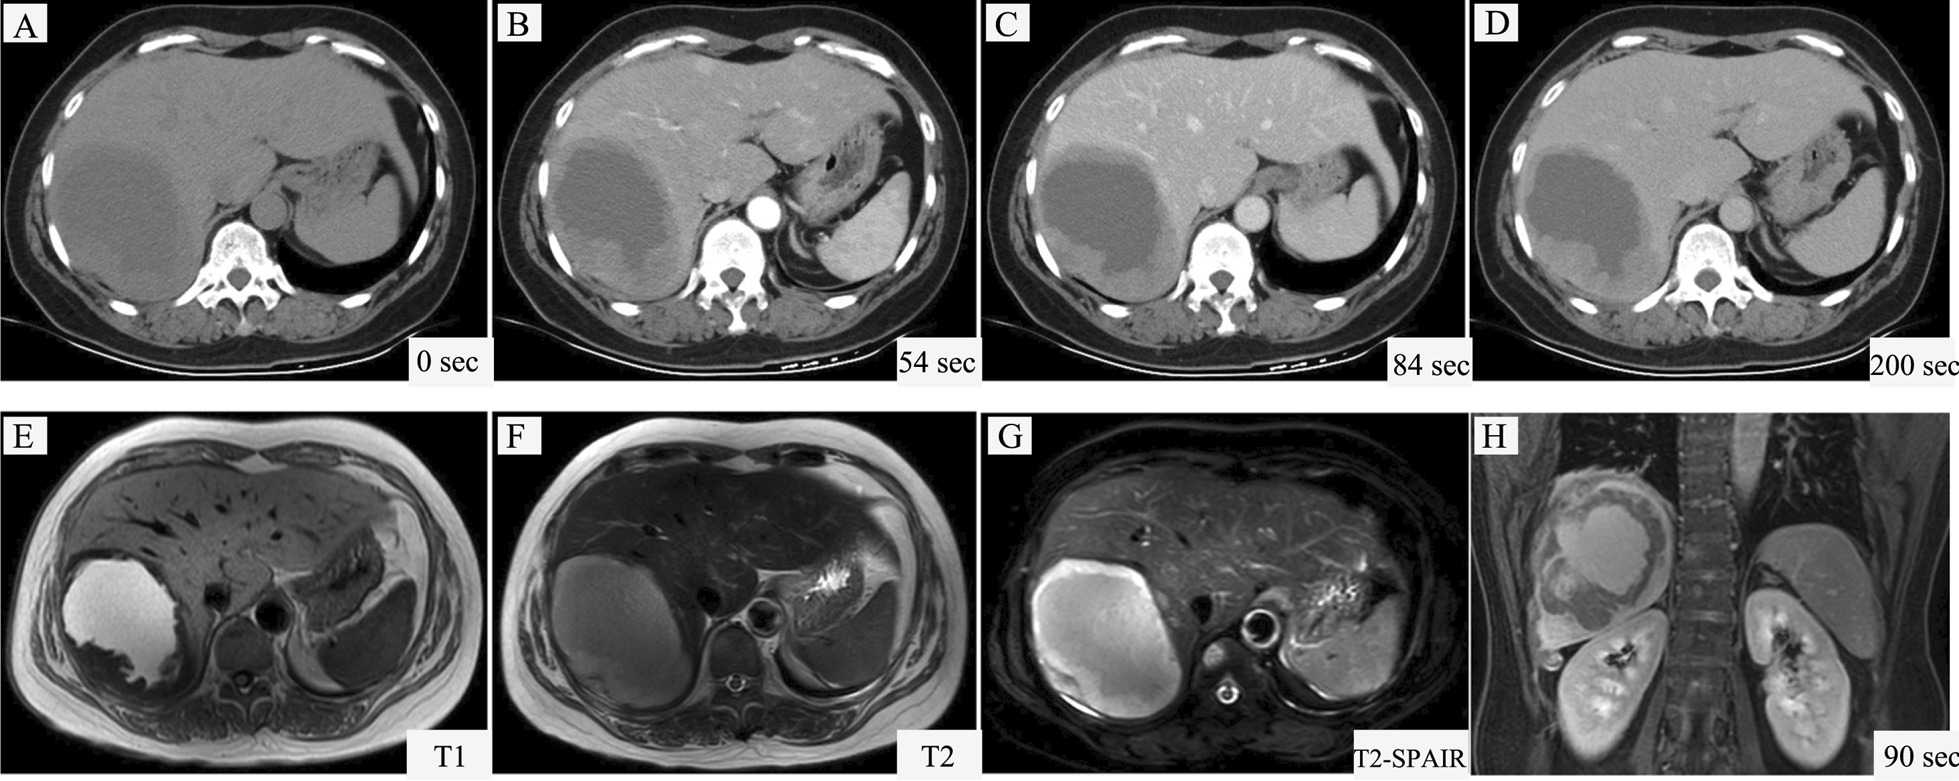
Imaging findings of the patient. Computed tomography (CT) scan showed an ill-defined, hypodense mass in the right liver lobe extending to the diaphragm (A) with moderate enhancement in the periphery of the mass at 54 s (B), 84 s (C) and 200 s (D) after intravenous injection of contrast. Magnetic resonance imaging (MRI) demonstrated an elliptic mass in the right liver lobe with an increased signal on both T1- (E) and T2- (F) images; On T2- SPAIR sequence the mass showed high signal indicating haemorrhage within the mass (G); H Post-contrast axial T1-weighted sequence showed the capsule of the right lobe of liver was unevenly thickened and enhanced but no obvious enhancement was visible inside the tumor
